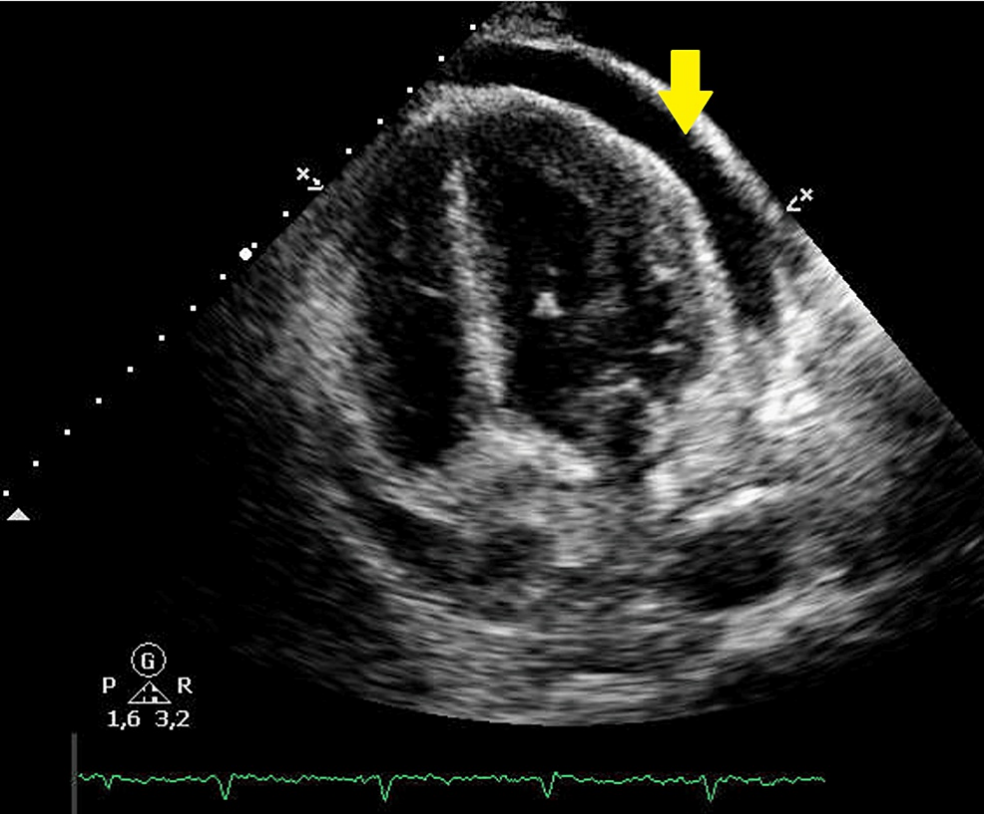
Pericardial effusion on echocardiogram. The arrow points to the large pericardial effusion visible with this imaging method.
